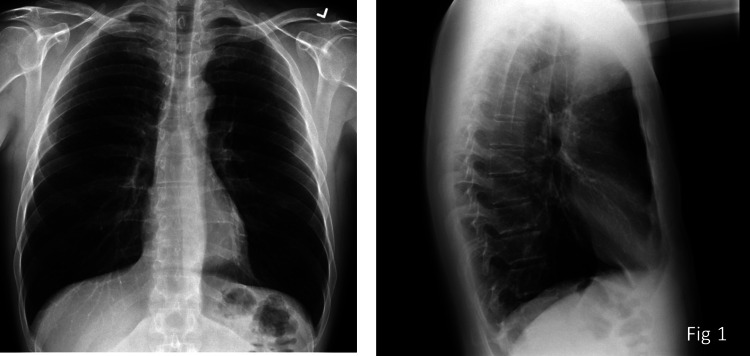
X-ray images of healthy chest and lungs AP view (left-hand side image) and lateral (right-hand side image) views. Image source: Reference no. [10]
